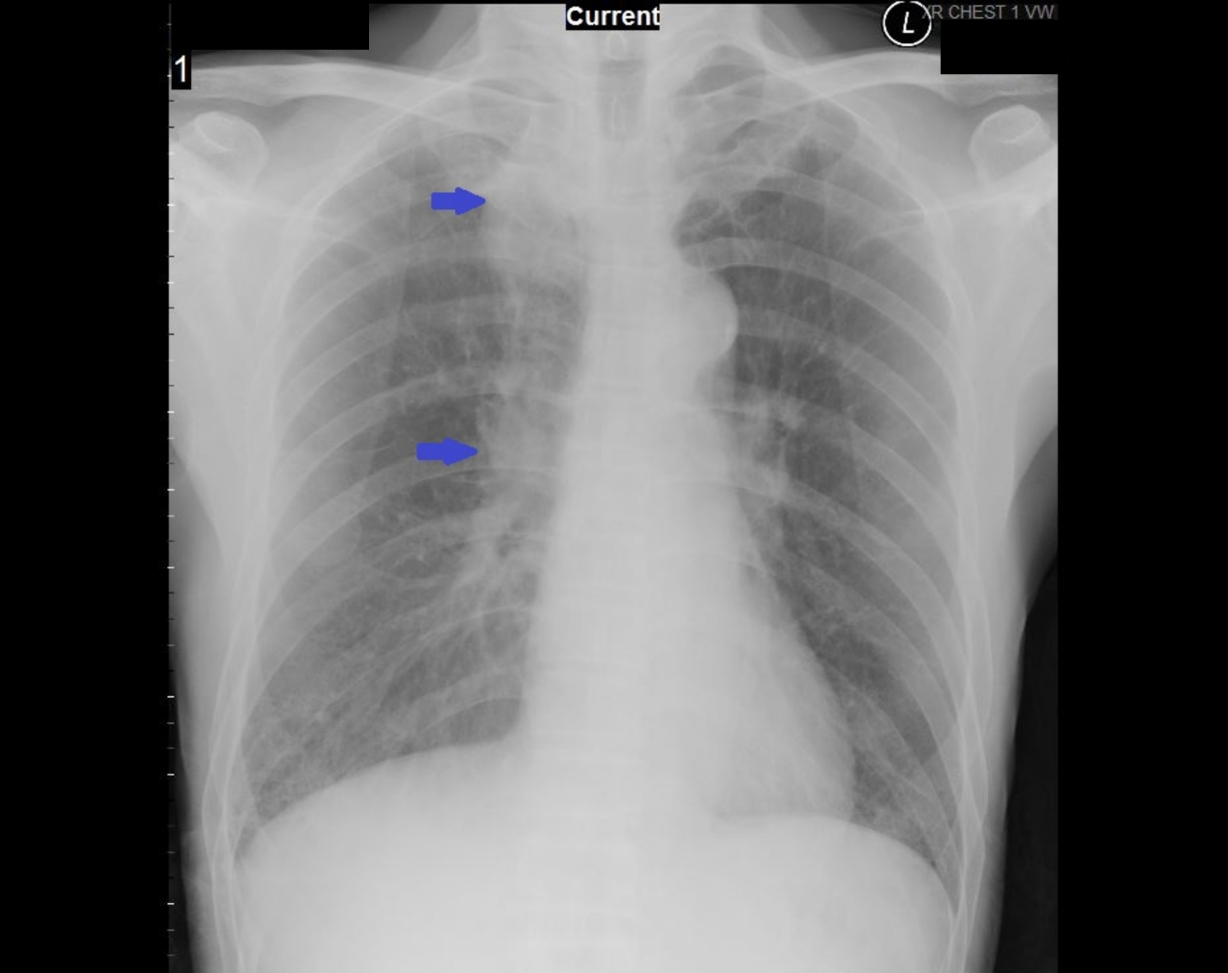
The chest X-ray showing postoperative changes of the right lung, pulmonary fibrosis, and borderline enlarged lower mediastinal lymph nodes adjacent to the distal esophagus.
